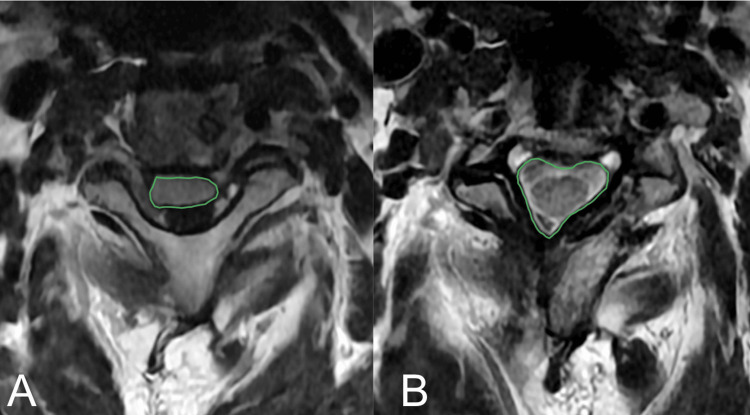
(A) Preoperative and (B) postoperative axial MRI images showing good decompression and reconstitution of spinal canal volume and CSF signal around the entirety of the cervical spinal cord. The green lines denote the spinal cord CSA capturing the dural sac only. CSA: Cross-sectional area
